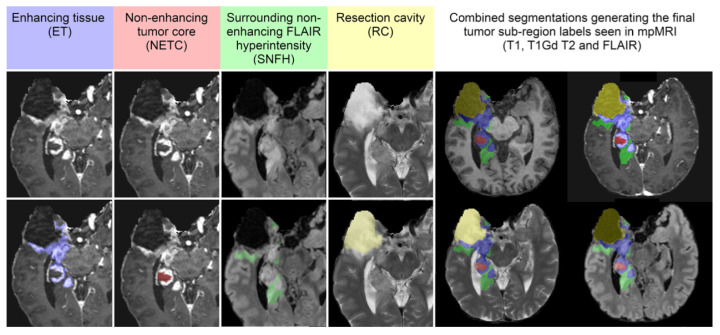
Sub-regions considered in the 2024 BraTS post-treatment glioma challenge include the enhancing tissue (blue), the non-enhancing tumor core (red), the surrounding non-enhancing FLAIR hyperintensity (green), and the newly introduced label for the resection cavity (yellow). Figure adapted from [34], licensed under the CC BY 4.0: https://creativecommons.org/licenses/by/4.0/ (accessed 15 October 2024).
